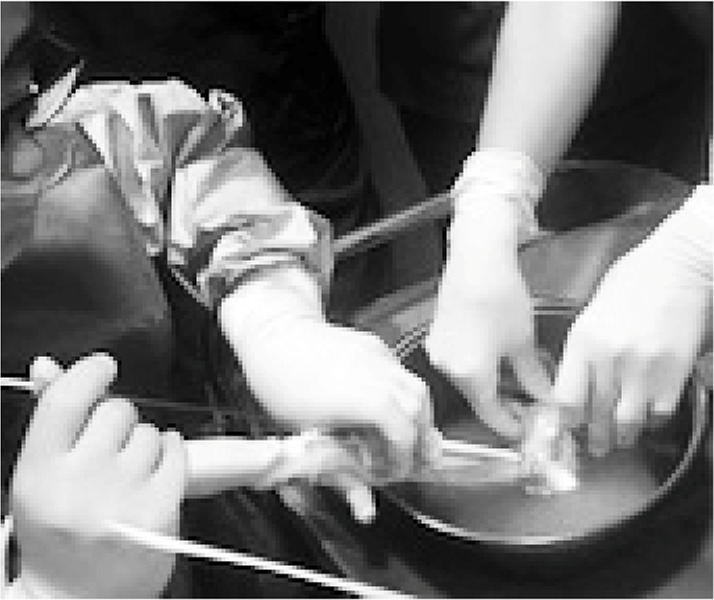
Follicular aspiration using vaginal ultrasound probe
